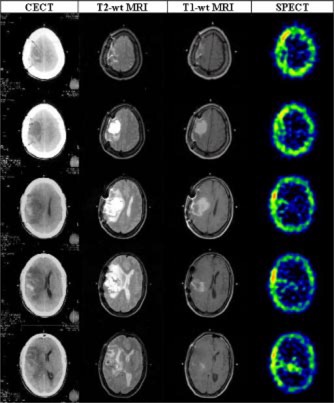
(Color) Corresponding axial sections of the CECT, *T*2‐weighted, *T*1‐weighted MR and SPECT images.
